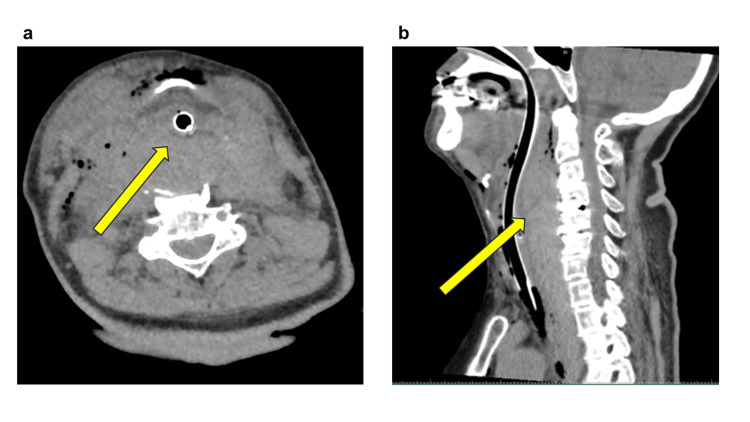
Computed tomography (CT) after emergency intubation CT images demonstrate a large retropharyngeal hematoma compressing the upper airway following anterior cervical discectomy and fusion (arrow). (a) Axial CT; (b) Sagittal CT
